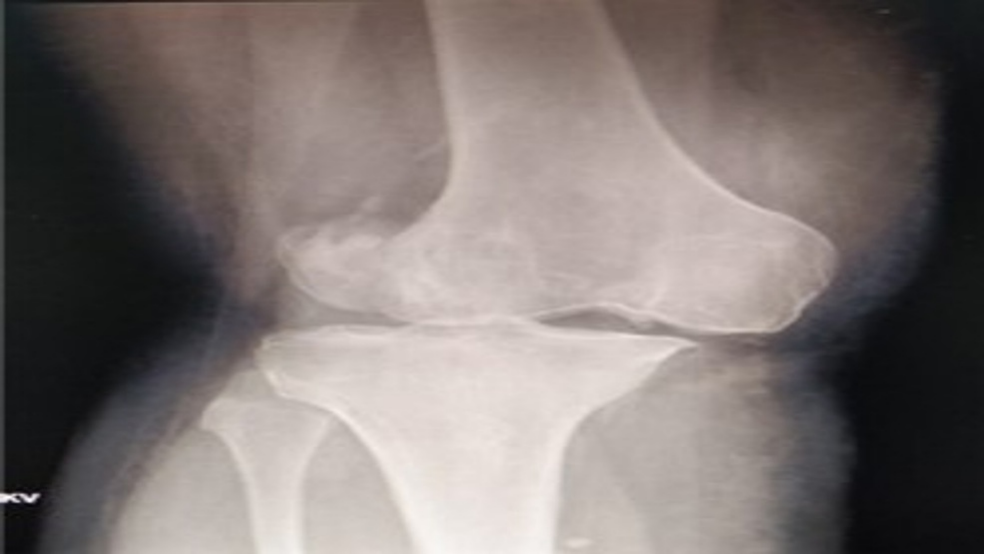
X-ray of the knee: anteroposterior view.
